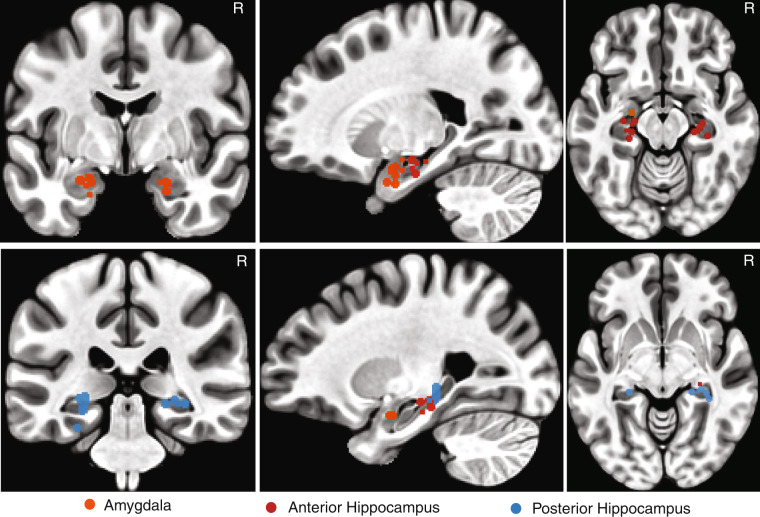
Electrode locations in the MNI space. The recording sites of each patient were estimated according to the pre-implantation T1-weighted MRI and post-implantation CT. All sagittal slices are from the left hemisphere. R: right.
